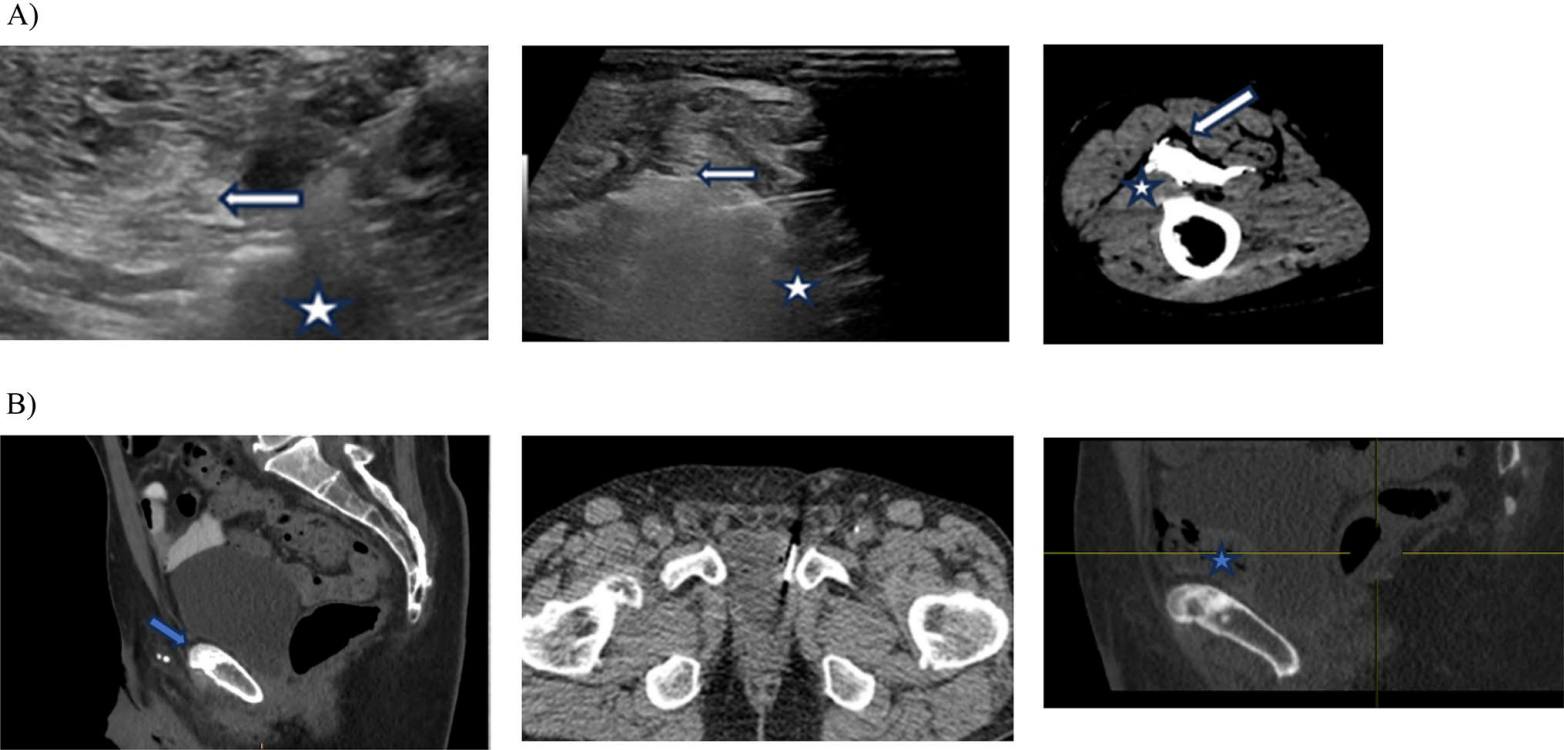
A Ultrasound showing a metastatic lymph node (white star) in the popliteal fossa and the adjacent neurovascular bundle (white arrow). Real time ultrasound image showing the hydrogel injection which creates a snowstorm appearance. The neurovascular bundle is partially obscured by the gel. Post procedure confirmatory CT demonstrating adequate displacement between the lymph node and the neurovascular bundle. B CT image of a sclerotic osseus metastasis in the pubic symphysis (blue arrow) abutting the bladder. SpaceOAR injection using CT guidance as US was limited due to osseous structures. Post procedure CT reconstruction demonstrating SpaceOAR hydrogel (blue star) displacing the bladder away from the osseous metastasis
